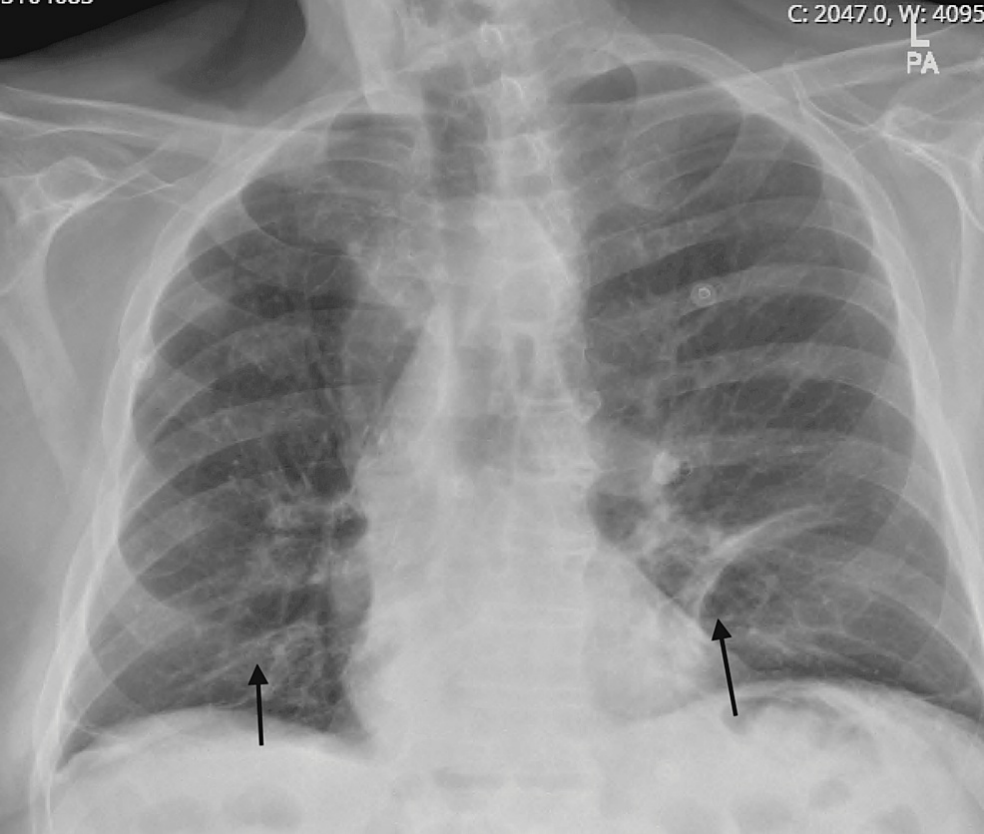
Chest x-ray with subsegmental atelectasis in the lung bases in the setting of acute respiratory failure.
